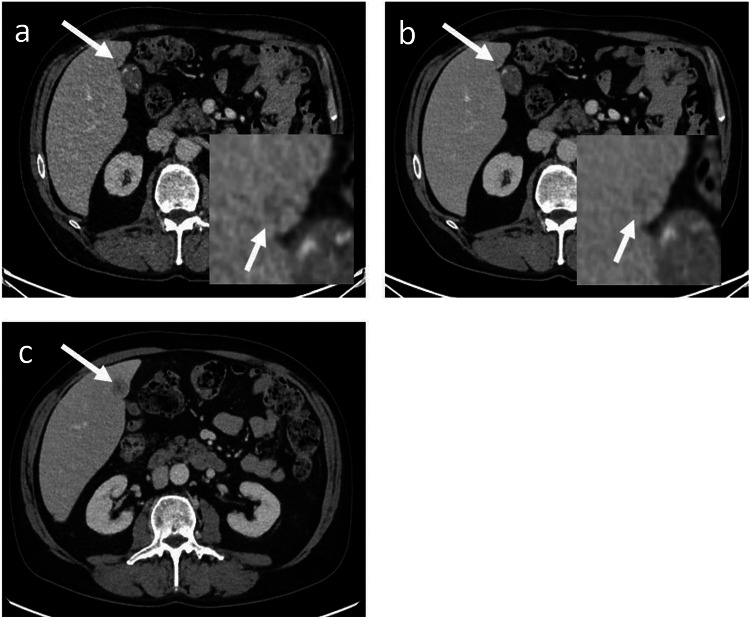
A contrast-enhanced CT image obtained with ASiR-V (a) and DLIR-H (b) showing the same hypoattenuating metastasis, magnified in the right lower corner (white arrows). Both readers detected the lesion on DLIR-H and missed the diagnosis on ASiR-V, as did a third independent reader. CT image of the same patient two months later showing the growth of the lesion and confirming its malignancy (white arrow) (c)
